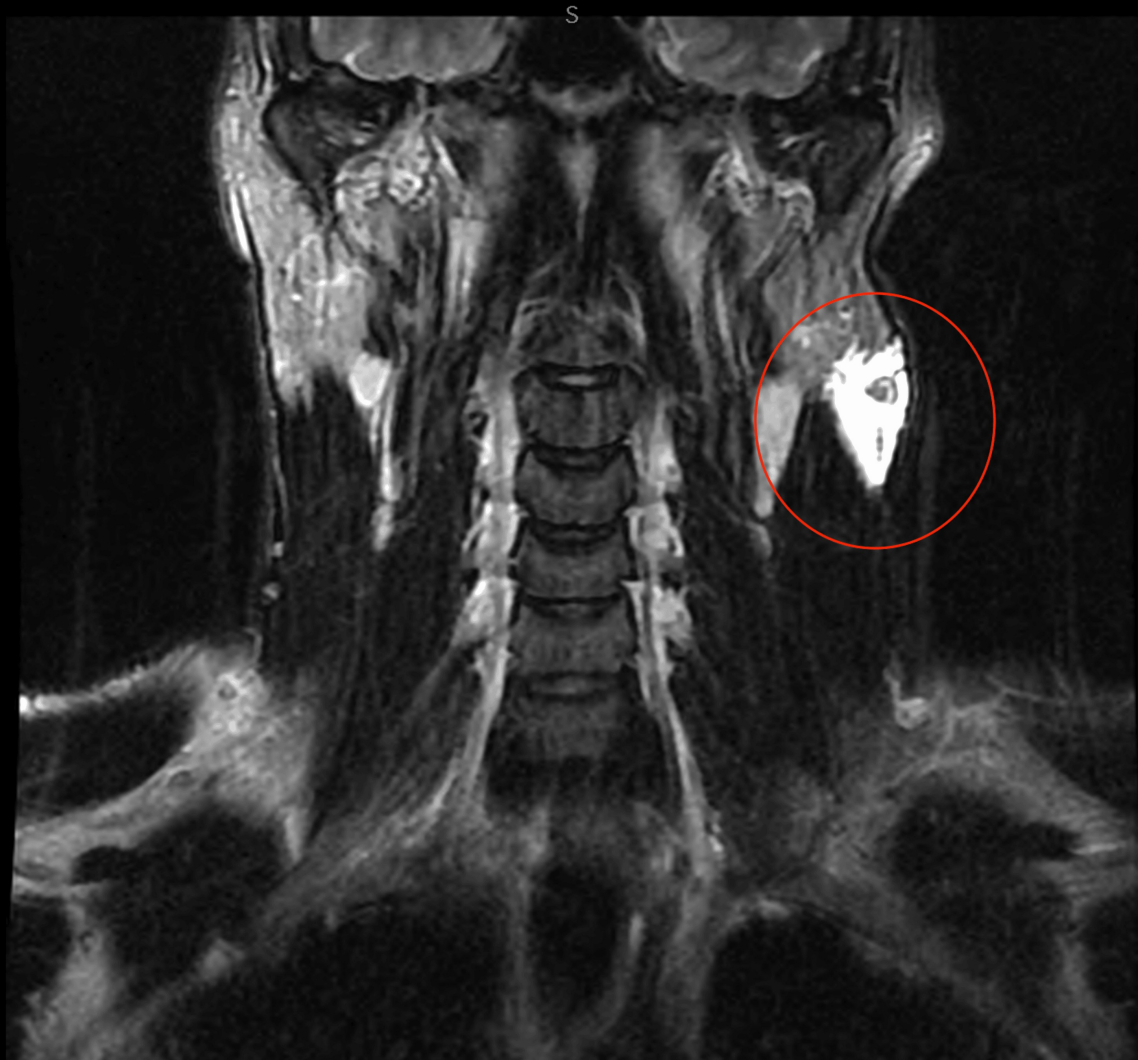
Axial T2-weighted MRI showing a multiseptated, hyperintense lesion (circled in red) with intralesional fat and minimal enhancement.
